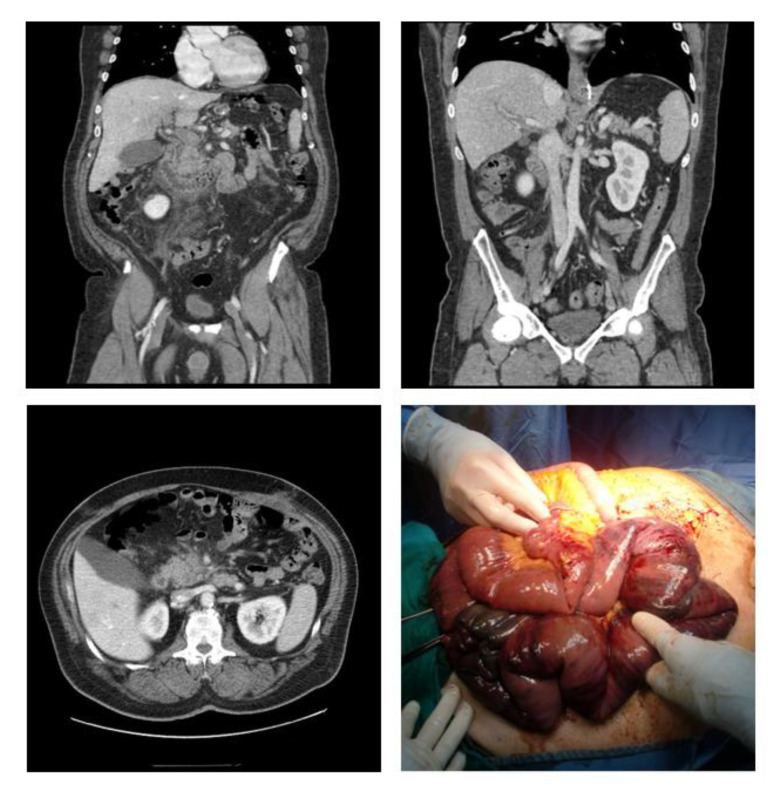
Case of portal vein thrombosis after biliopancreatic diversion; CT scan showing extended PVT associated with bowel ischemia, and related intraoperative findings. PVT: Portal Vein Thrombosis.
